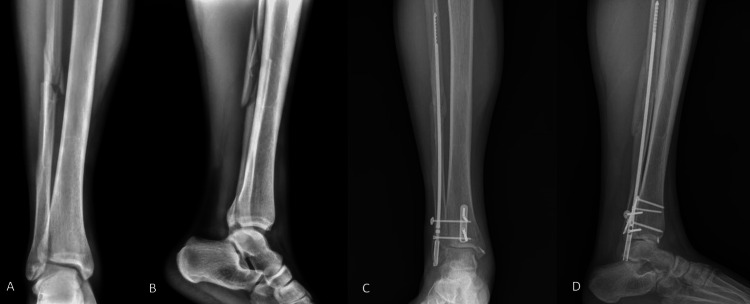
Nail fixation (A) Preoperative anteroposterior radiograph, (B) preoperative lateral radiograph, (C) postoperative (one year follow-up) anteroposterior radiograph, (D) postoperative (one year follow-up) lateral radiograph
